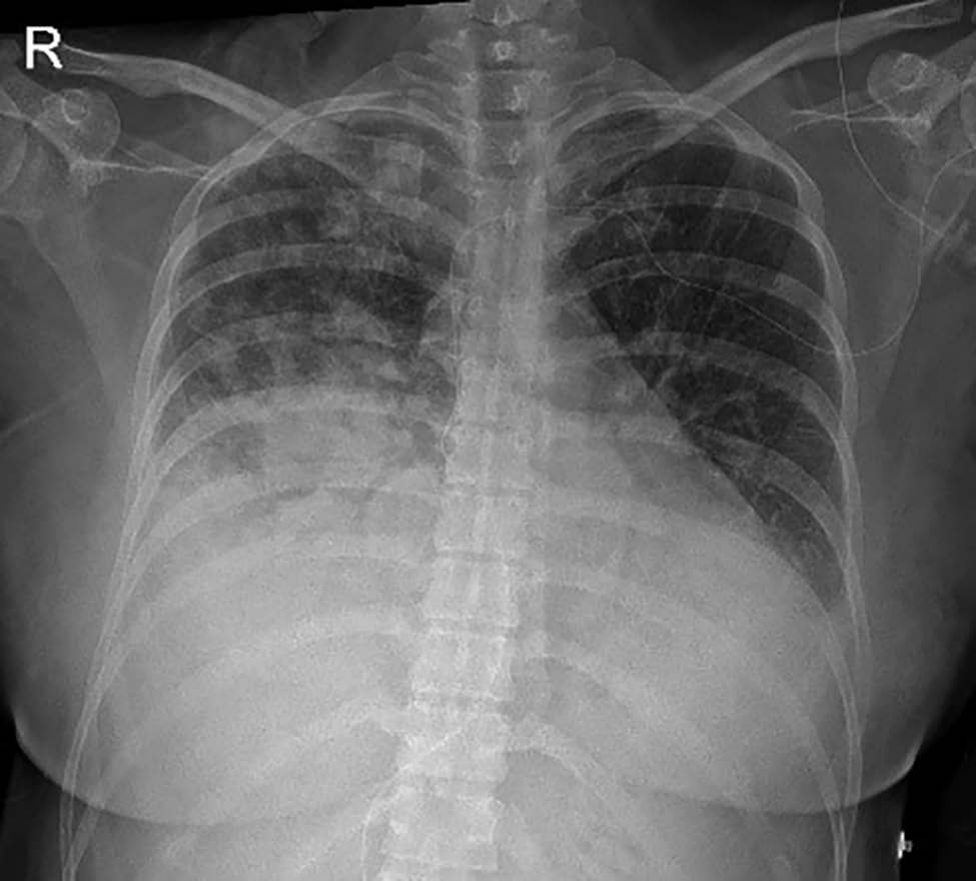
Chest X-ray POD 1 decreased RUL haziness, remaining haziness at RUL. POD = postoperative day.
